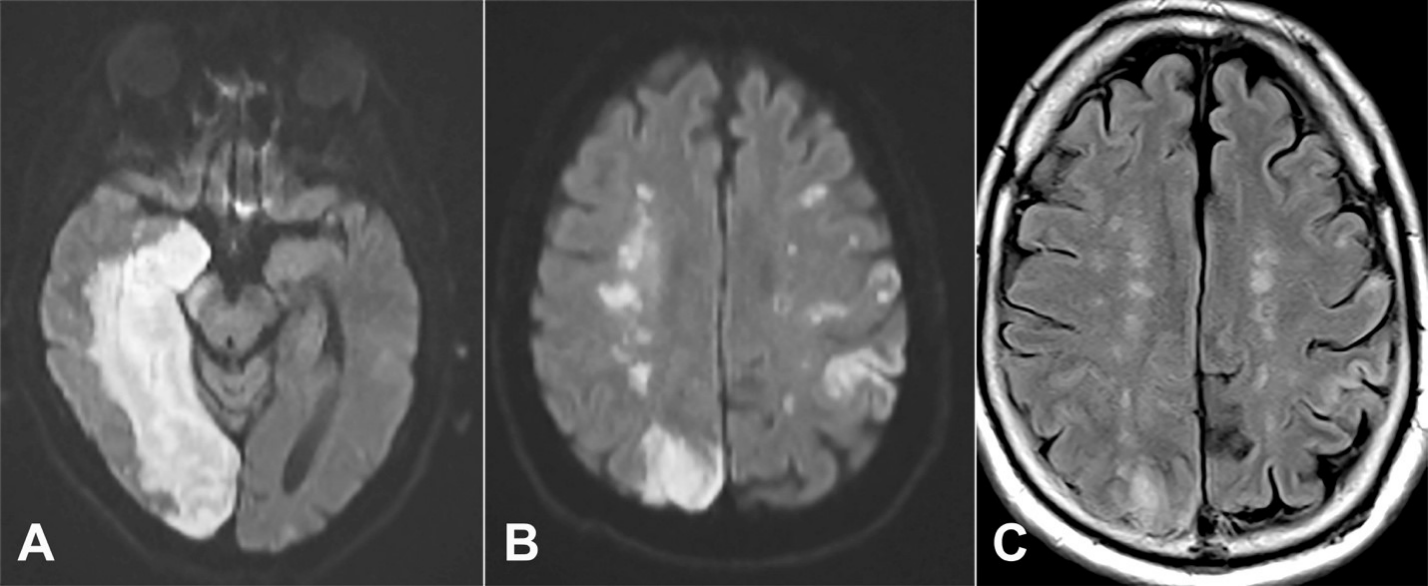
Magnetic resonance imaging from hospital day 4. A - the acute large right posterior cerebral artery infraction is seen affecting the right medial temporal lobe and occipital lobe on the diffusion weighted imaging; B - at the upper border of the right parieto-occipital cortical infarction, one can see a string of bright areas in the deep centrum semiovale on the right side representing acute watershed infarction on this diffusion weighted image. Acute cortical strokes are seen in the left hemisphere; C - the fluid attenuated inversion recovery (FLAIR) scan confirms the deep watershed infarctions bilaterally, seen as higher signal intensity on this imaging. The visualization on FLAIR suggests the infarctions are over 6 hours old. Diffusion weighted imaging remain positive for 7-15 days.
